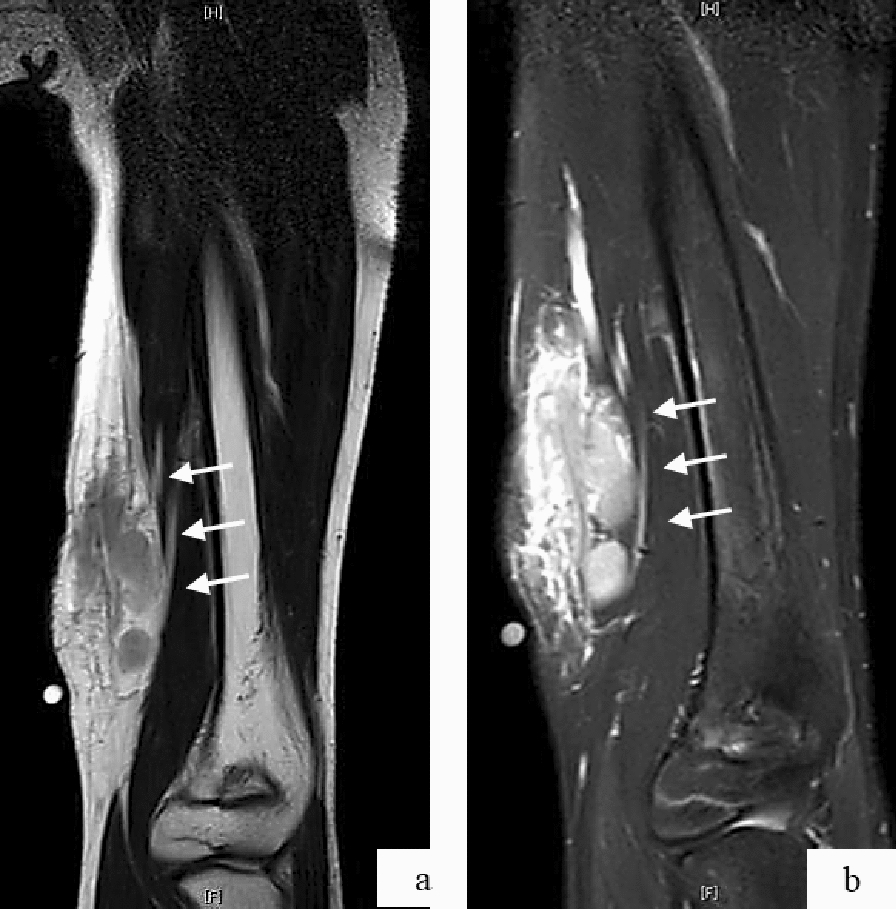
Magnetic resonance imaging of the left proximal arm. a T2-weighted coronal MR image reveals the presence of a subcutaneous lesion in the medial aspect of the left distal arm (white arrow). b Fat suppression T2-weighted coronal MR image shows the presence with a lesion of complex intermediate signal intensity (white arrow)
